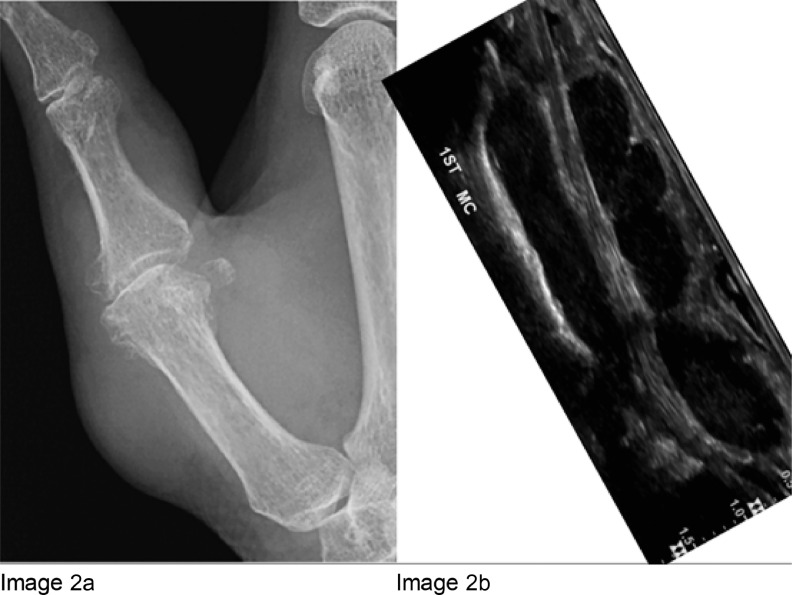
Image 2a: PA radiograph of the right thumb. There is soft tissue swelling over the dorsolateral aspect of the thumb without any underlying bony scalloping or erosion. There are degenerative changes at the metacarpophalangeal articulation of the thumb. Image 2b: Ultrasound of the thumb. The ultrasound displays a multilobulated hypoechoic lesion measuring up to 6cm, surrounding the right flexor pollicis longus (FPL) tendon. The FPL tendon is the hyperintense linear structure.
